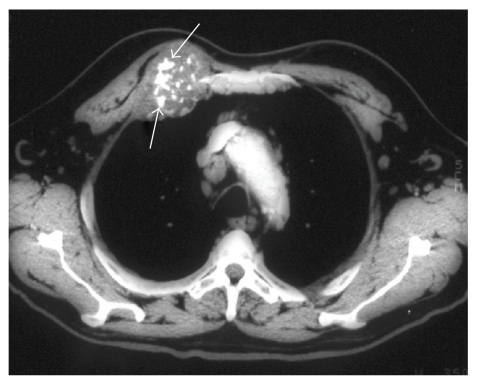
Typical CT appearance of an anterior chest wall chondrosarcoma arising from the chondrosternal junction, demonstrating prominent chondroid matrix mineralization resulting in a characteristic flocculent or “popcorn” pattern of calcification [6].
